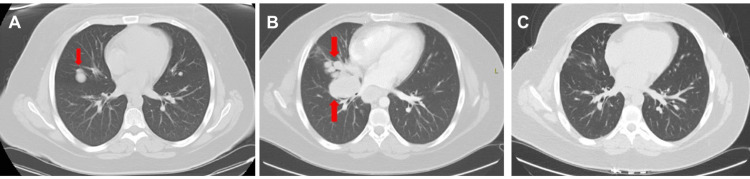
CT of the chest showing (A) initial lung nodules in 2011, (B) progression of the lung nodules in 2015, and (C) complete remission of the lung nodules in 2023
